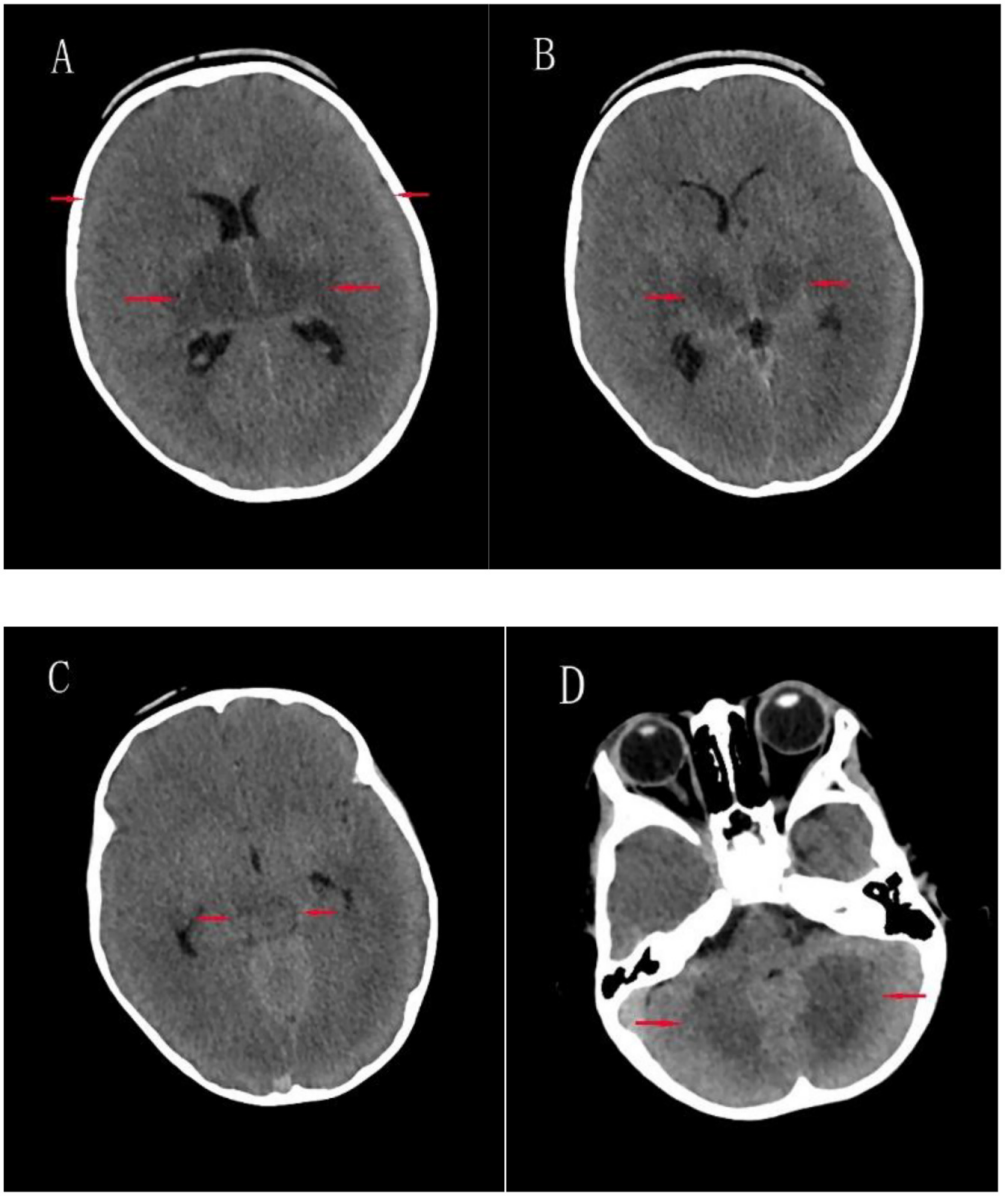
Axial brain CT images of case 2. (A) Bilateral cerebral hemispheric swelling with effacement of sulci and fissures, blurred gray-white matter differentiation, and mildly hypodense areas in the bilateral basal ganglia; (B) mild hypodensity in the bilateral thalami; (C) Mild hypodensity in the upper midbrain; (D) Patchy mild hypodensities in the bilateral cerebellar hemispheres.
